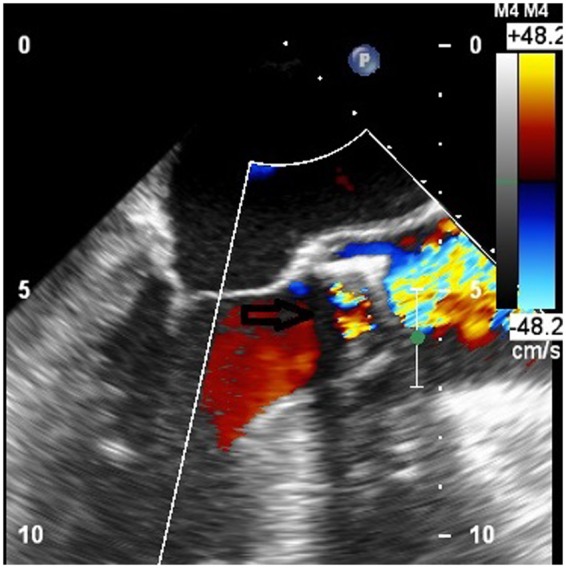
Transoesophageal echocardiogram mid-oesophageal long-axis view showing turbulent flow through the bioprosthetic aortic valve (arrow pointing).
